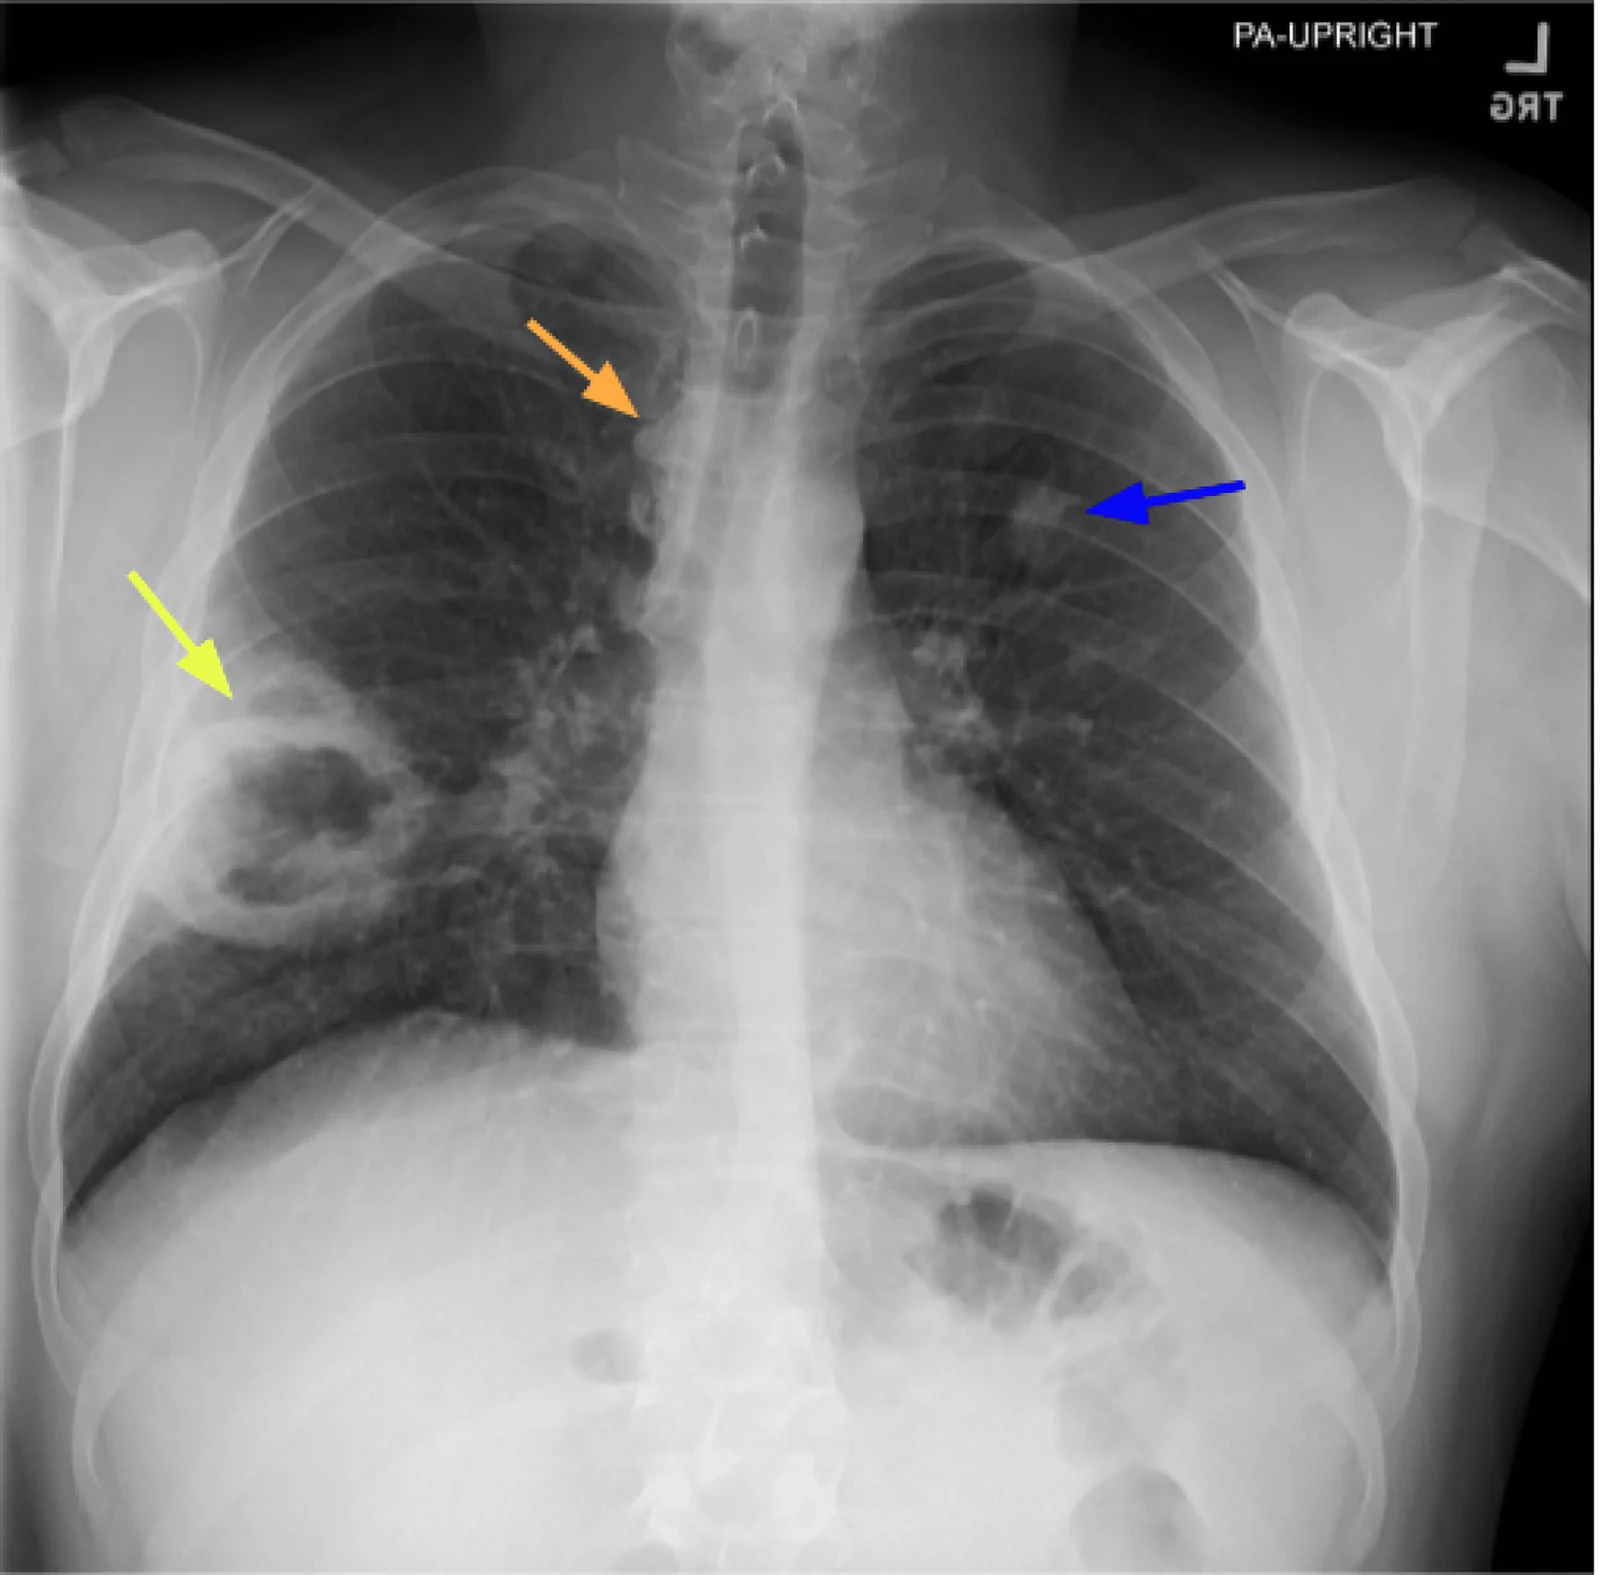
Posteroanterior chest radiograph demonstrating a large cavitary lesion centered within the superior segment of the right lower lobe, with possible extension toward the right middle lobe (yellow arrow). Additional small focal nodular opacities are present in the right upper lobe (orange arrow) and left upper lobe (blue arrow).
